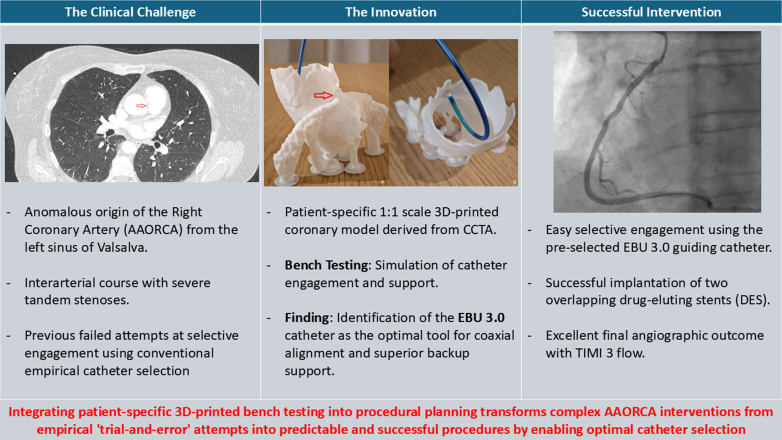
3D-Guided PCI in Complex AAORCA (Left) The Clinical Challenge: Symptomatic anomalous right coronary artery with failed conventional engagement attempts. (Middle) The Innovation: Patient-specific 1:1 scale 3D-printed model derived from CCTA for preprocedural bench testing. (Right) Successful Intervention: Identification of EBU 3.0 as the optimal catheter, leading to successful revascularization with drug-eluting stents and TIMI flow grade 3. Conclusion: 3D-printed bench testing transforms empirical “trial-and-error” into a predictable and successful procedural strategy. 3D = 3-dimensional; AAORCA = anomalous aortic origin of the right coronary artery; CCTA = coronary computed tomography angiography; PCI = percutaneous coronary intervention.
